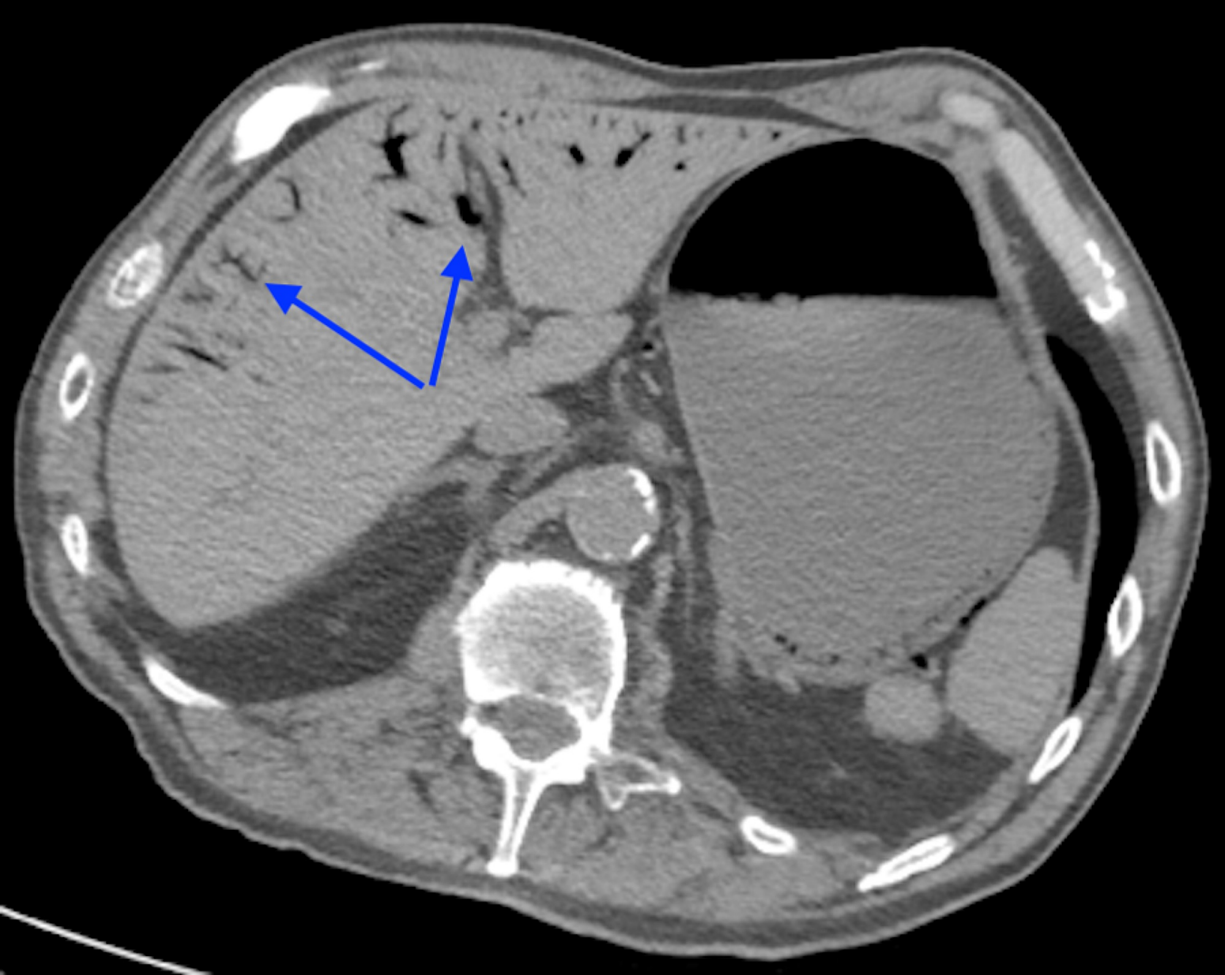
Axial CT scan of the abdomen demonstrating gas in the portal and perigastric veins (arrows). CT, computed tomography
